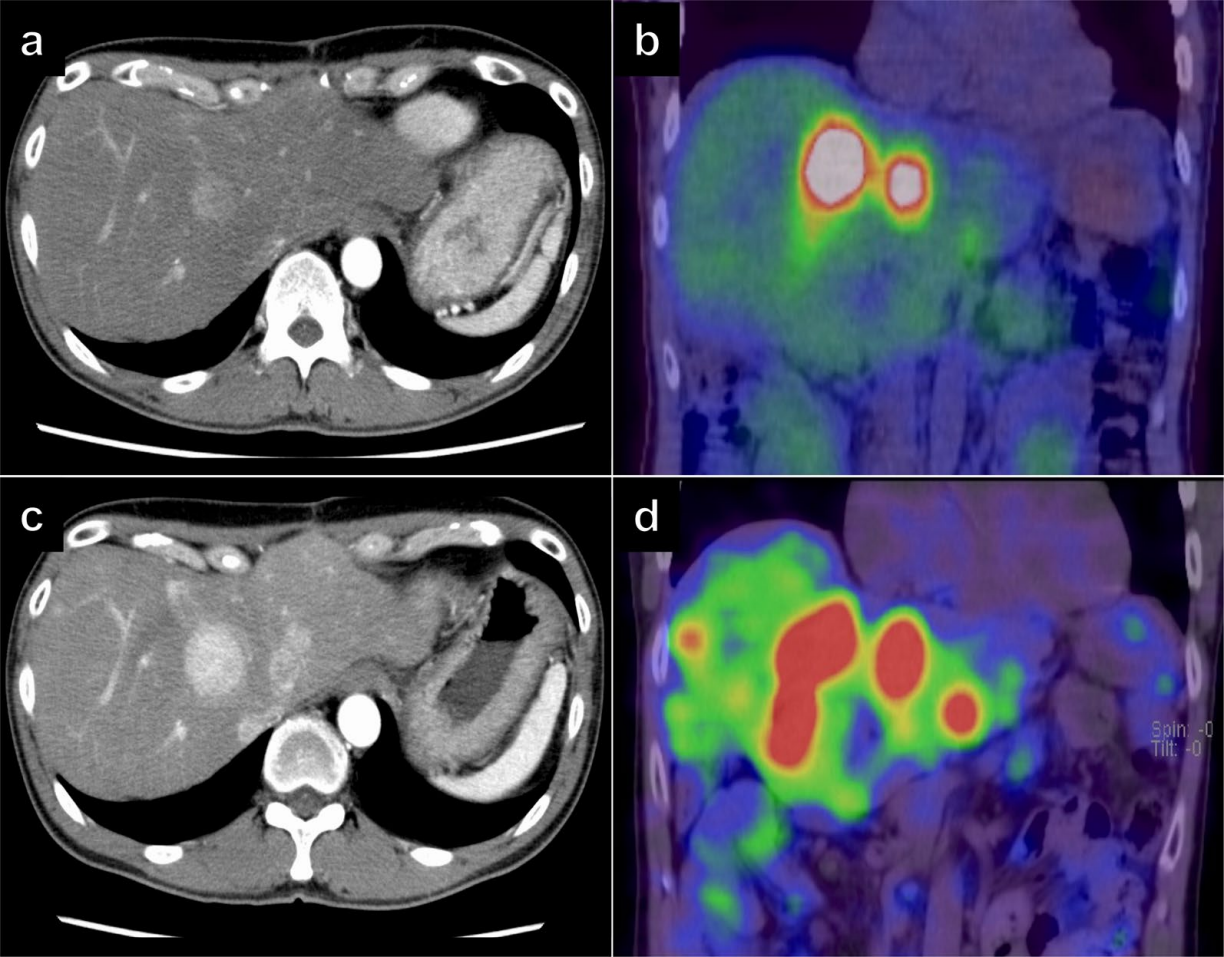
Third recurrence. Contrast-enhanced computed tomography revealed multiple liver metastases (a), and somatostatin receptor scintigraphy confirmed the abnormal accumulation in the tumor (b). Despite administering lanreotide, the tumor progressed (c and d)
